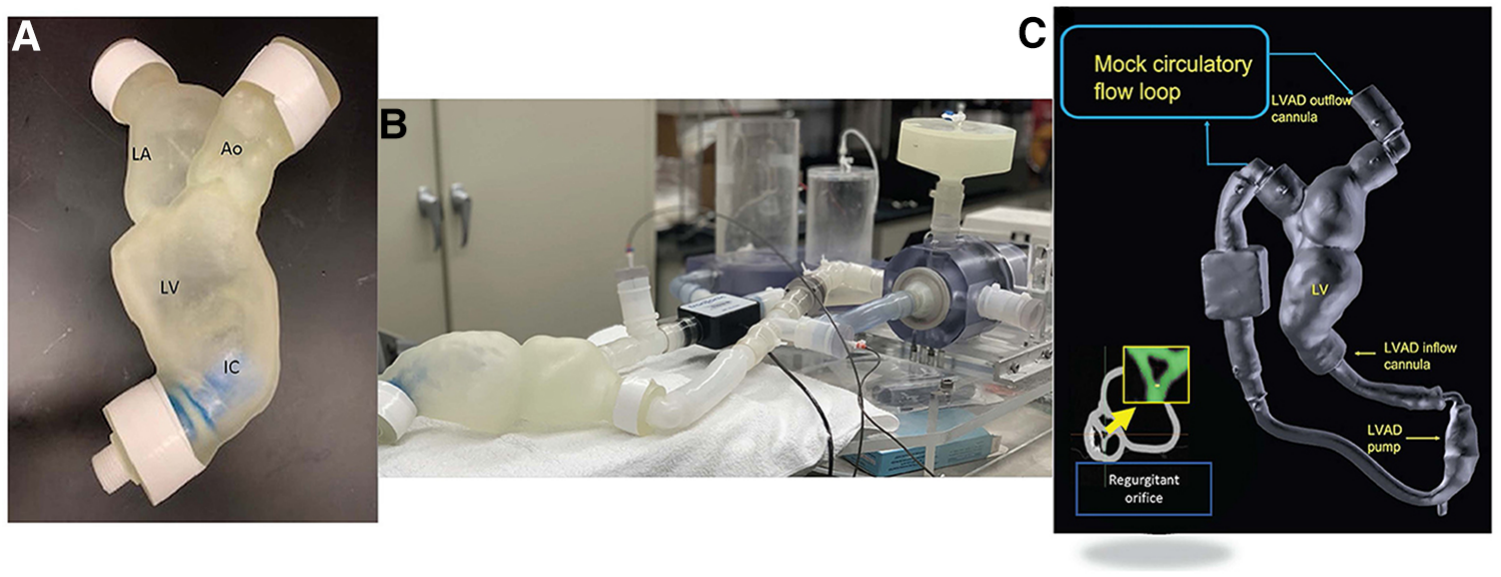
(A) 3d printed model of patient anatomy (atria and left ventricle), reconstructed from CT data, (B,C) model implemented in a mock circulatory loop, enabling study of changes in the system due to changes in VAD settings or other parameters. This set up can also be used to validate a computational model involving the same anatomy and boundary conditions (29).
